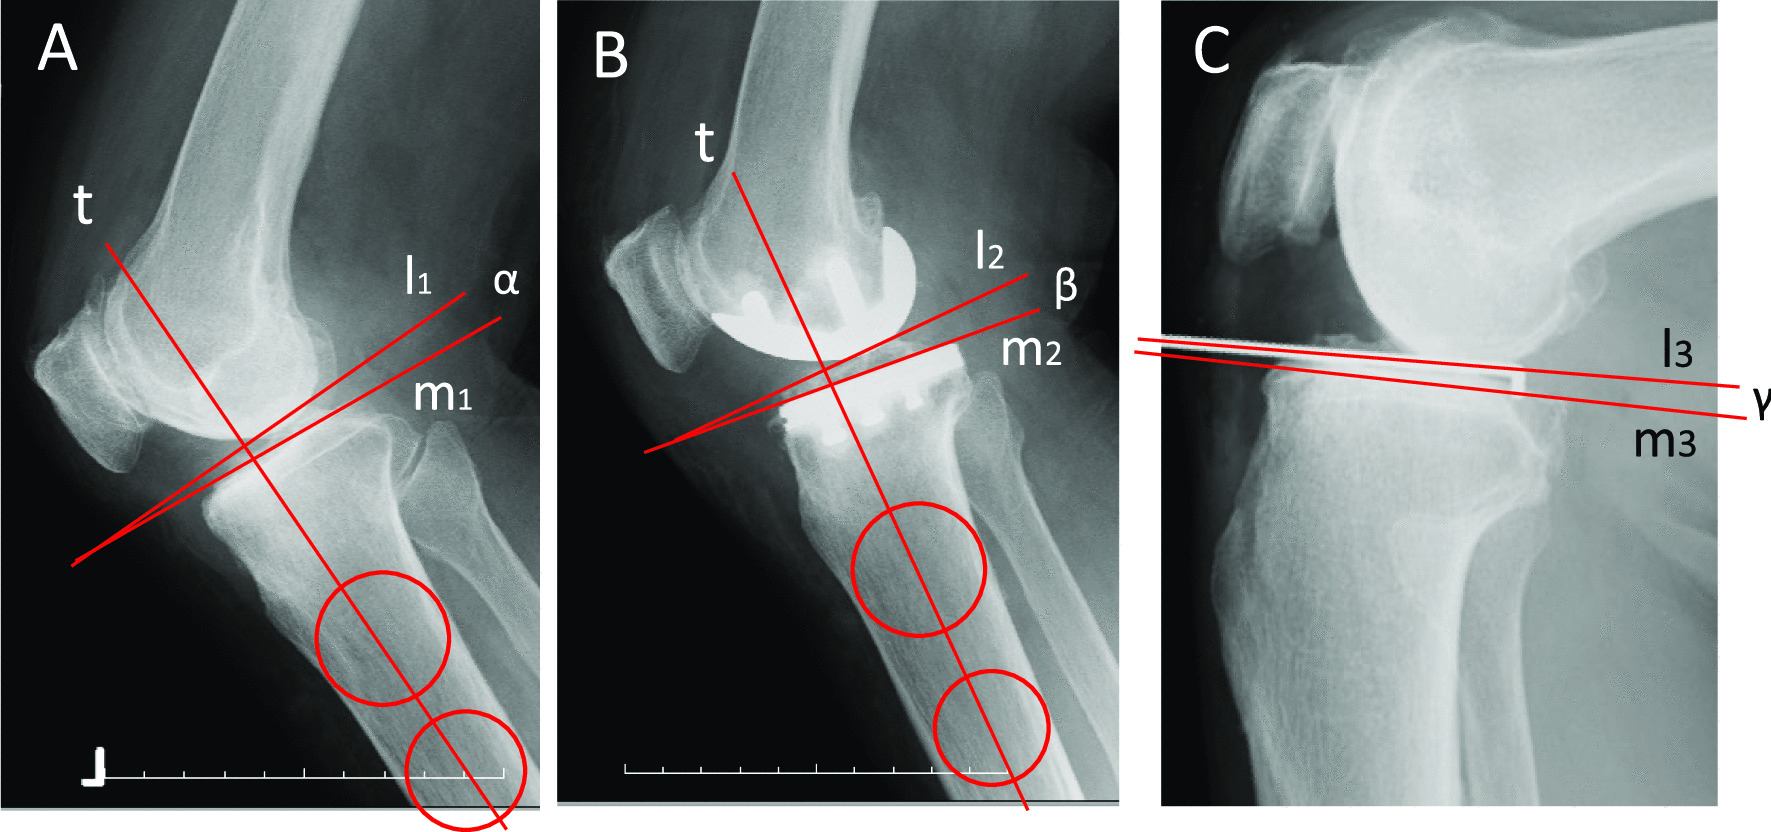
Measurement of the angles on preoperative, intraoperative, and postoperative lateral knee radiography. Preoperative bony PTS (α) and postoperative implant PTS (β) on a lateral radiograph were measured according to a method previously reported (A, B). On the intraoperative lateral knee radiograph, an angle (γ) between the native bony MTP (m_3_) and the cartilage MTP (l_3_) was measured (C)
